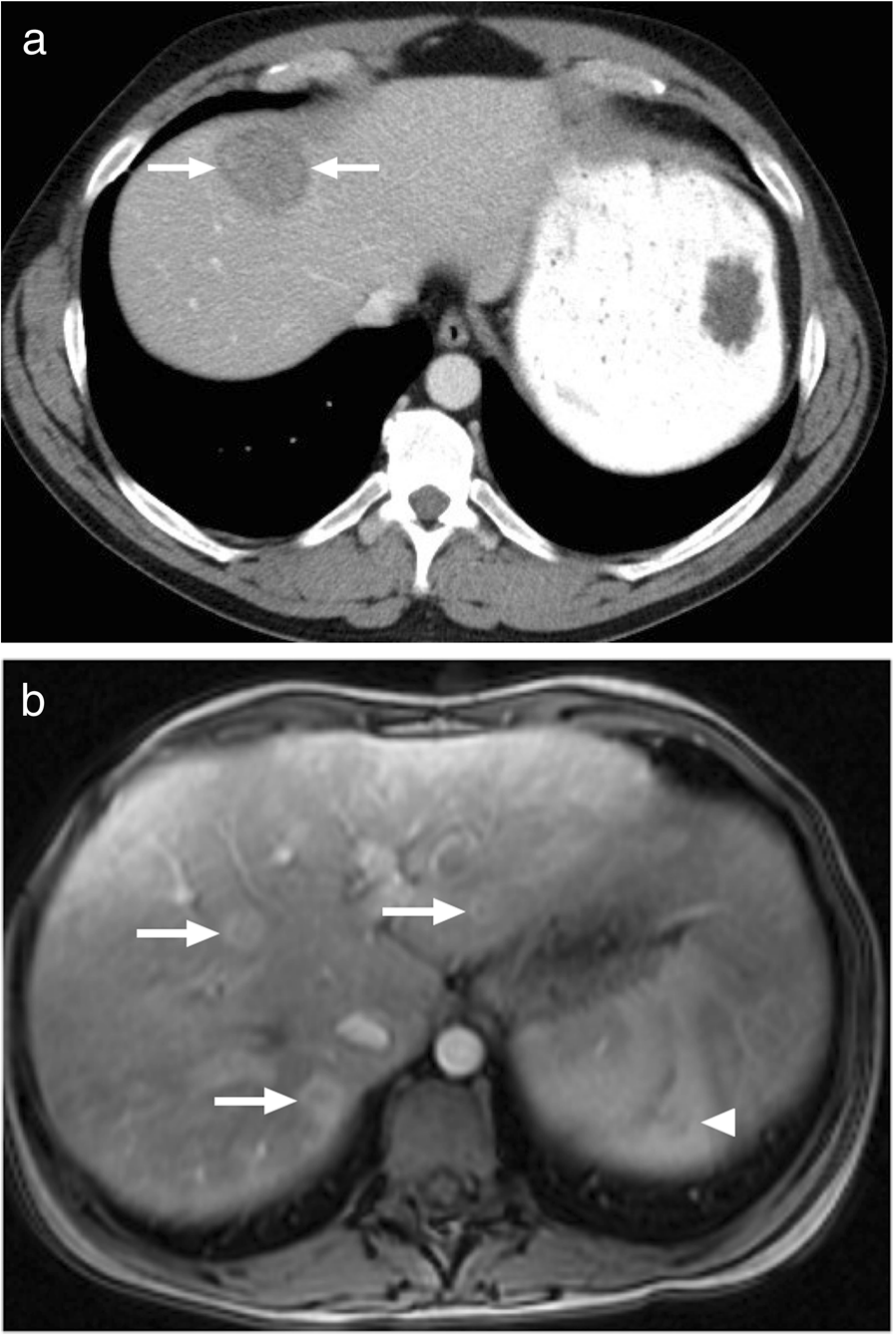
Images of two different patients with pathologically proven hepatic Bartonella granulomas. a Axial plane postcontrast CT image of a 45-year-old male demonstrates predominantly hypodense solid mass (arrows), with central heterogeneity, located in the liver dome. Surgical resection of this lesion confirmed Bartonellosis. b A 13-year-old girl presented with abdominal pain and referred for MRI with liver lesions detected on ultrasound. Axial plane postcontrast arterial phase image demonstrates avidly ring-enhancing liver masses (arrows) and similar lesions in the spleen (arrowhead) which were more evident on portal venous phase image (not shown). These lesions were concerning for hypervascular metastasis. Acute titers were positive for Bartonella and the patient recovered uneventfully
